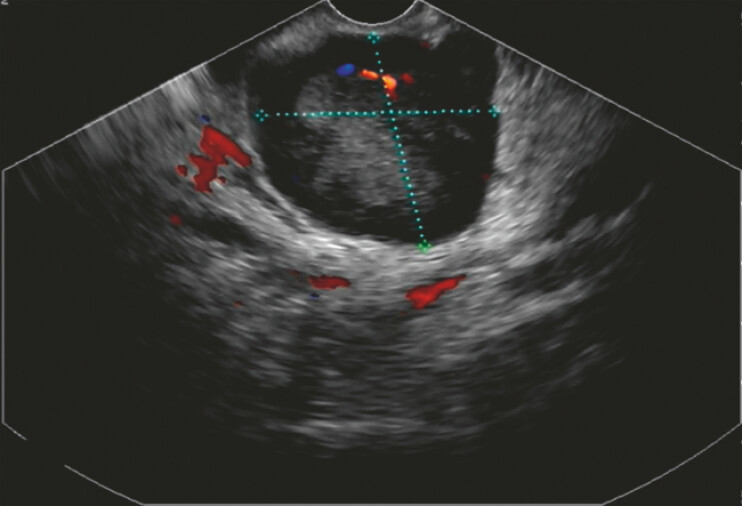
Endoscopic ultrasound revealed a hypoechoic lesion measuring 38.14 × 35.75 mm.
